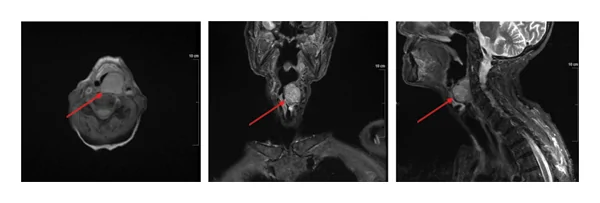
Magnetic resonance imaging revealing a large 3.2 × 2.5 × 3 cm low‐density mass arising in the region of the left posterior supraglottic larynx, some projecting into the supraglottic larynx, narrowing the airway. Red arrows indicate the hypopharyngeal mass.
